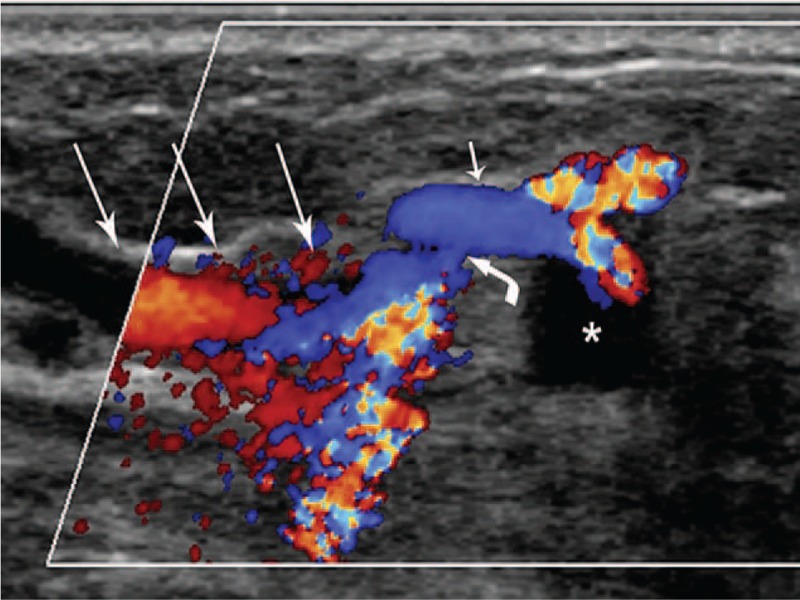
Another color Doppler imaging section showed the pseudoaneurysm (asterisk) with associated arteriovenous fistula, the artery feeding the pseudoaneurysm (short arrow), the concomitant vein (long arrow), and the fistula (bent arrow) communicating with the feeding artery and concomitant vein, suggesting pseudoaneurysm with associated arteriovenous fistula.
